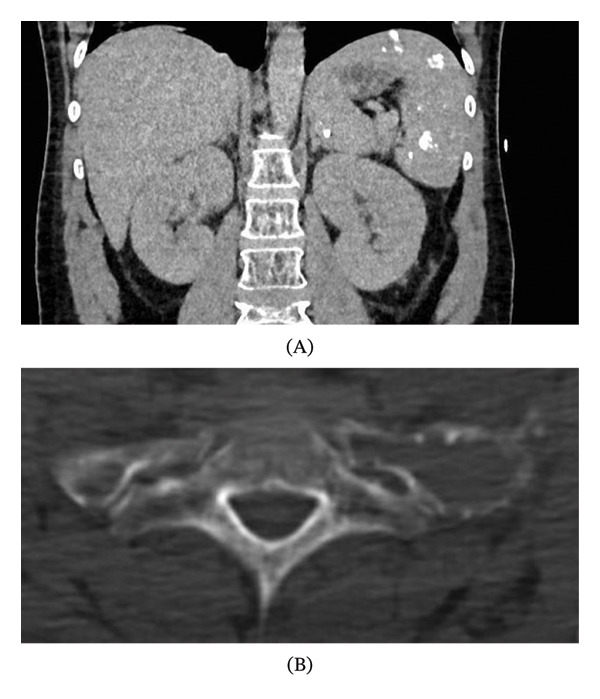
(A) Contrast‐enhanced CT of the abdomen, portal venous phase. The kidneys demonstrate edematous swelling and decreased corticomedullary differentiation. Incidentally noted are splenic calcifications. (B): Noncontrast CT of the cervical spine. A large lytic lesion of the left posterior first rib with a soft tissue component is noted.
